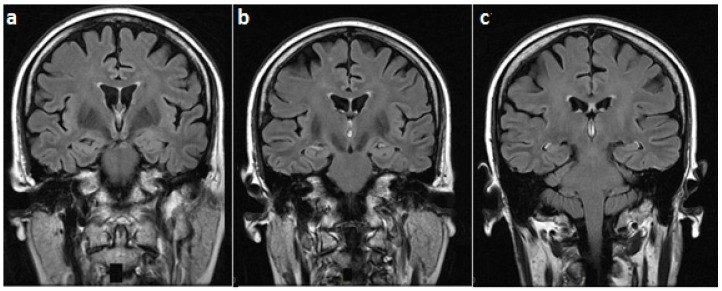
Fluid-attenuated inversion recovery brain magnetic resonance (MR) images (coronal view), at the level of (a) mammillary bodies, (b) third ventricle, and (c) midbrain, showing a normal magnetic resonance imaging (MRI) signal in these regions.
